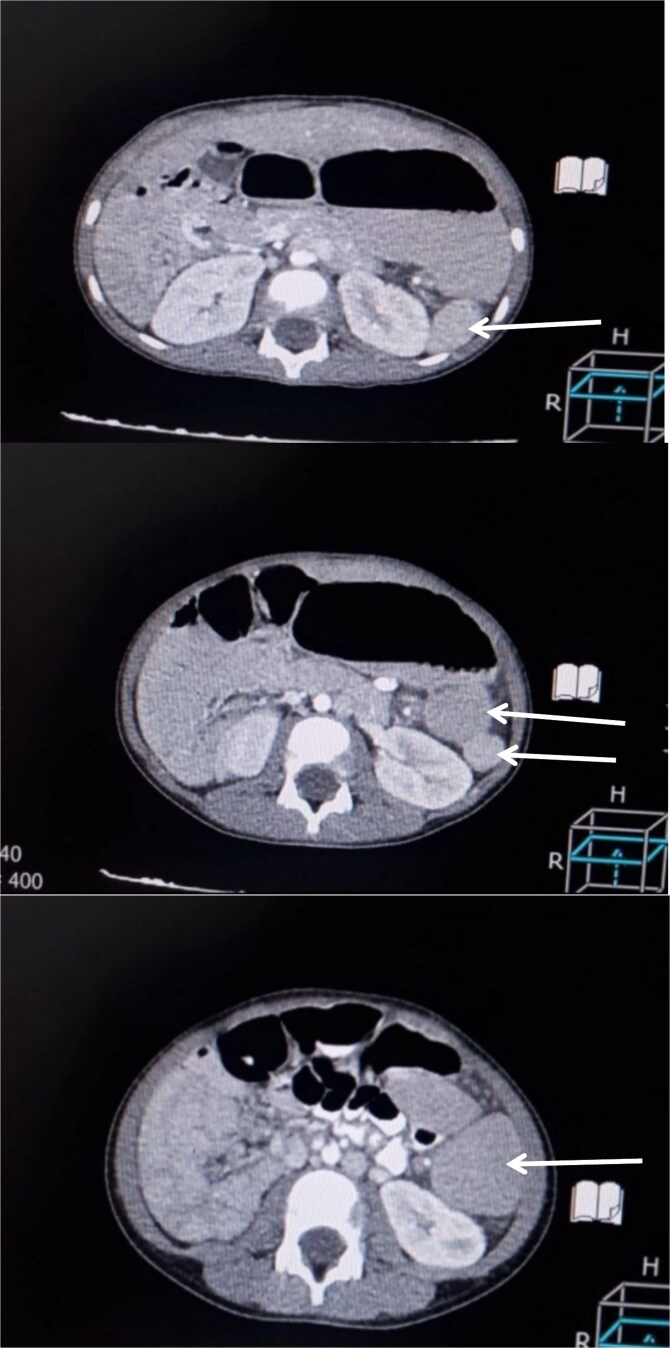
Contrast-enhanced CT scan of the abdomen demonstrating multiple spleen-like densities (arrows) can be seen in the left upper and lower quadrants of the abdomen.
